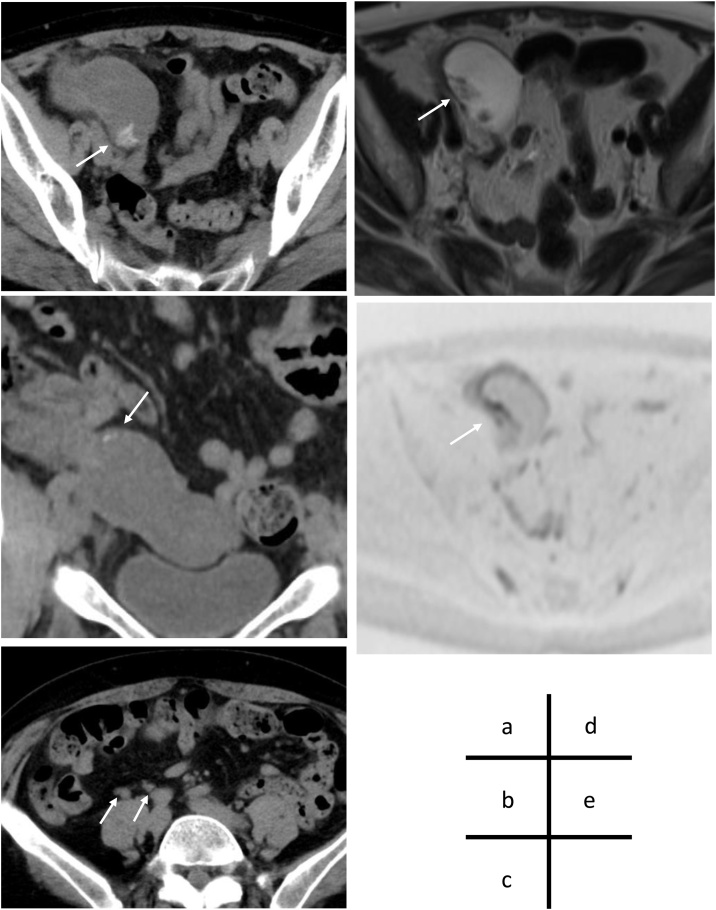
(a, b) Image of the appendix showing it was swollen up to 38 mm in diameter, and had a bright nodule inside (white arrow). (c) No ascites was observed; however, regional lymph nodes were enlarged (white arrow). (d, e) Magnetic resonance imaging (MRI) revealed a high-intensity nodular ridge on T2WI and accumulation on diffusion-weighted imaging (DWI, white arrow).
